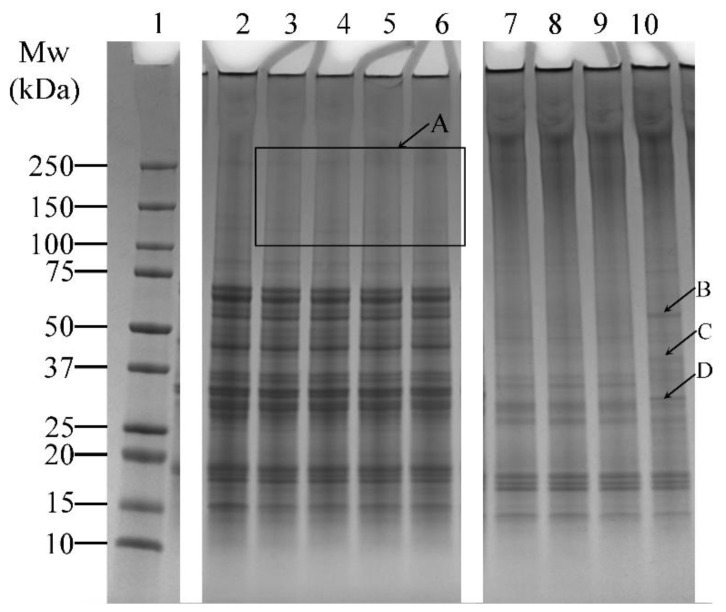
SDS-PAGE profile showing the cross-linking patterns of lupin and soy (LS) protein mixtures treated with laccase (LR) or transglutaminase (TG) at pH 7 and 30 °C under varying incubation times (1, 5, 10, and 20 h—H). Lane 1: Protein marker; Lane 2: LS-CT-1H Lanes 3–6: LS-LR-1H/5H/10H/20H; Lanes 7–10: LS-TG-1H/5H/10H/20H. CT—Control (non-enzyme-treated). A: Faint bands above 100 kDa in LR-treated samples, indicating limited cross-linking. B–D: New bands in TG-treated samples at 20 h, suggesting enhanced protein modifications. *Summary:*
